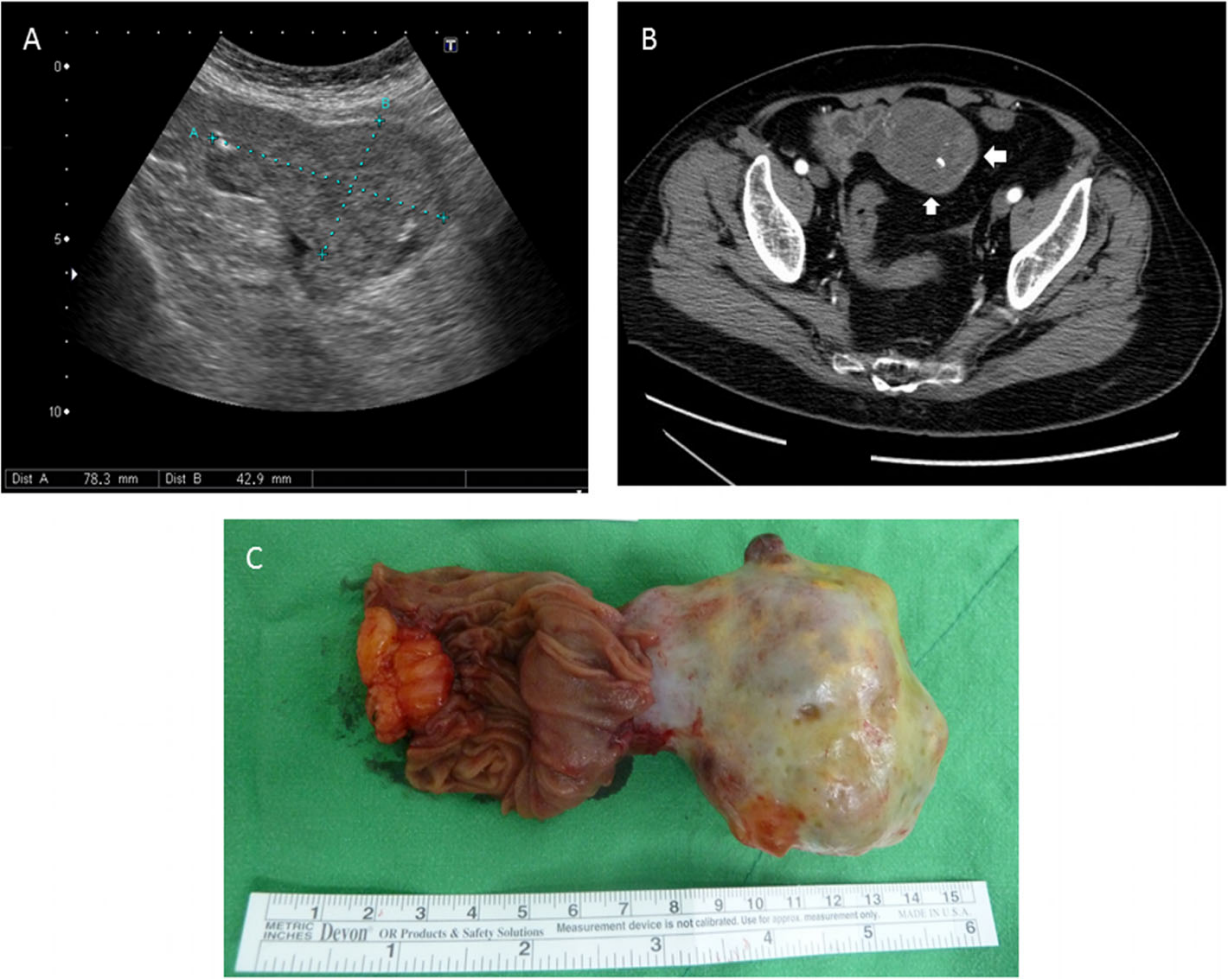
An ileal tumor. a, Transabdominal ultrasonography shows a 7.8 cm × 4.2 cm heterogeneous lesion over ileum. b, A large lobulated tumor with heterogeneous enhancement at the proximal ileum. c, A well-defined fleshy tumor at the proximal ileum was resected
